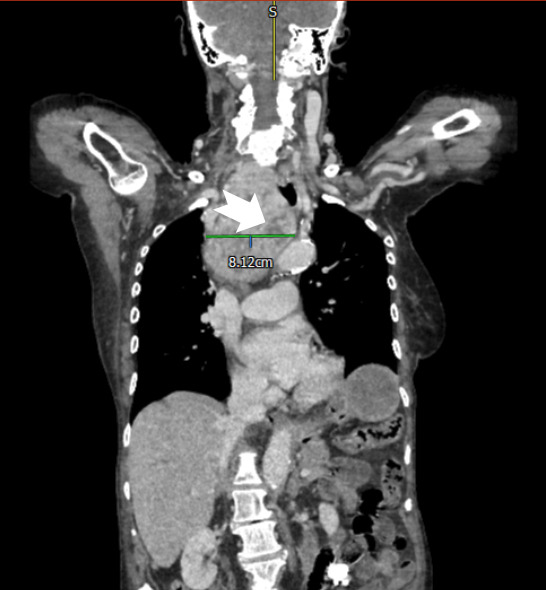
Coronal CT image of the chest and upper abdominal viscera showing a large left-sided irregular goitre (arrow) with normal appearance of the liver and spleen.
